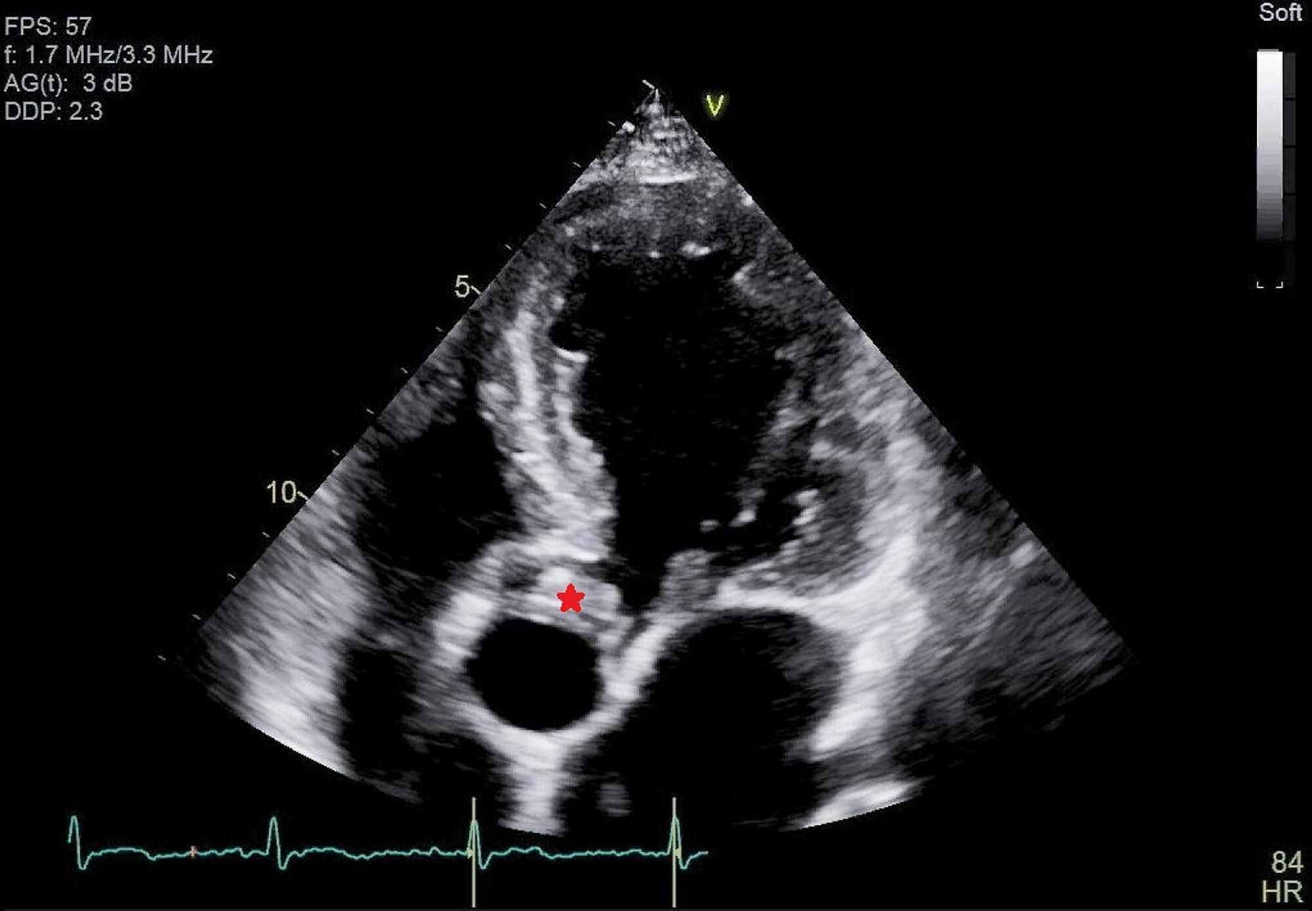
Transthoracic echocardiogram (apical five-chamber view) on admission showing a large aortic valve vegetation (red asterisk).
